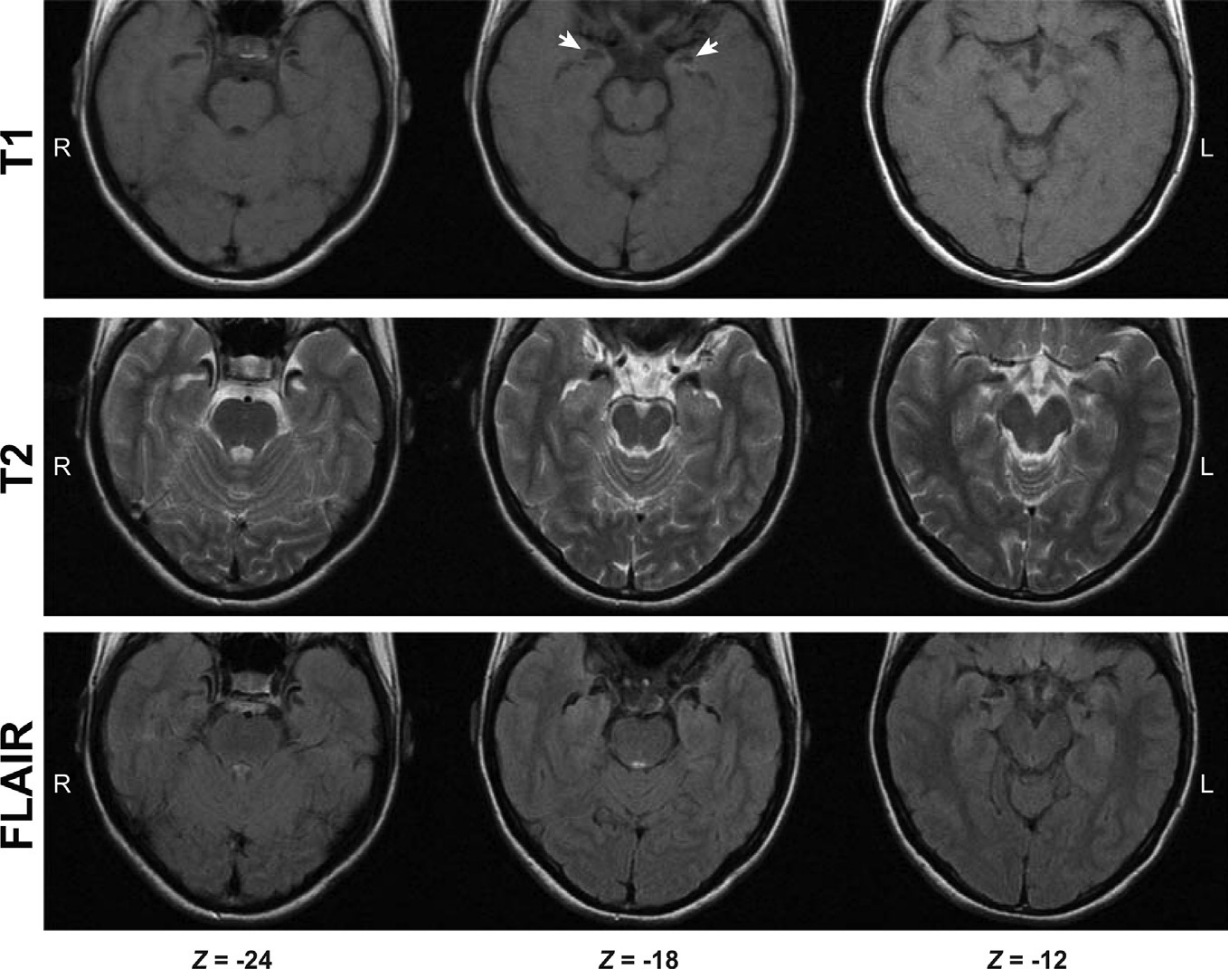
T1, T2, and FLAIR sequence MRI of SF. Images demonstrate bilateral amygdala lesions (arrowheads) as a result of symmetrical calcifications due to Urbach–Wiethe disease. Each column presents corresponding axial sections; from left to right at 24, 18, and 12 millimeters below the anterior commissure. Images are in radiological convention.
